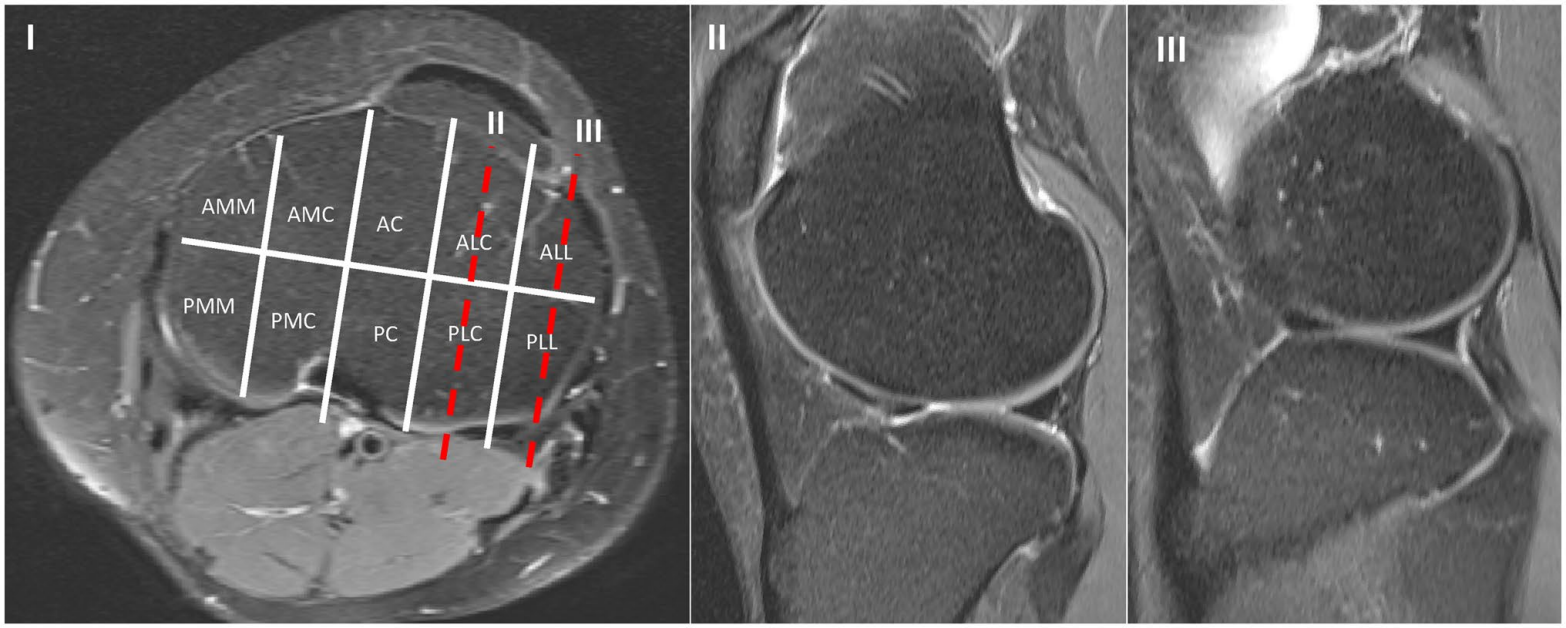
MRI of a 31 healthy woman. I axial slice of the MRI with applied 10 segment classification. The red dash line with the II shows the sagittal slice through the PLC segment, III shows the sagittal slice through the PLL segment. *ALL* antero latero-lateral, *ALC* antero-latero-central, *AC* antero-central, *AMC* antero-medio-central, *AMM* antero-medio-medial, *PLL* postero-latero-lateral, *PLC* postero-latero-central, *PC* postero-central, *PMC* postero-medio-central, *PMM* postero-medio medial; II sagittal section of the MRI's from I in the position of the red dash line marked II; III sagittal section of the MRI from I in the position of the red dashed line marked III
